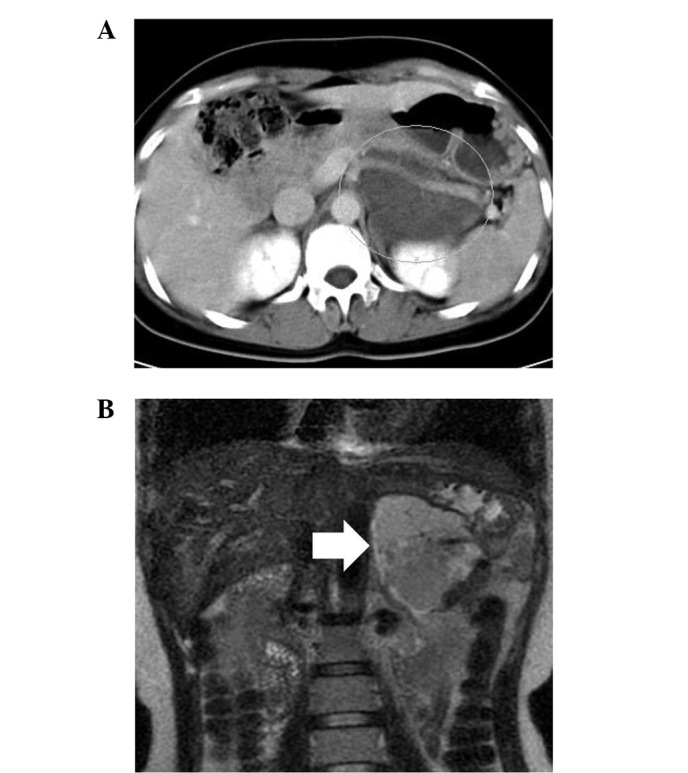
(A) An abdominal computed tomography scan showed a cystic lesion involving the splenic vein, as indicated by the circle. (B) Magnetic resonance imaging identified a high-attenuation, multilocular cyst with septa on T2-weighted imaging, as indicated by the arrow.
